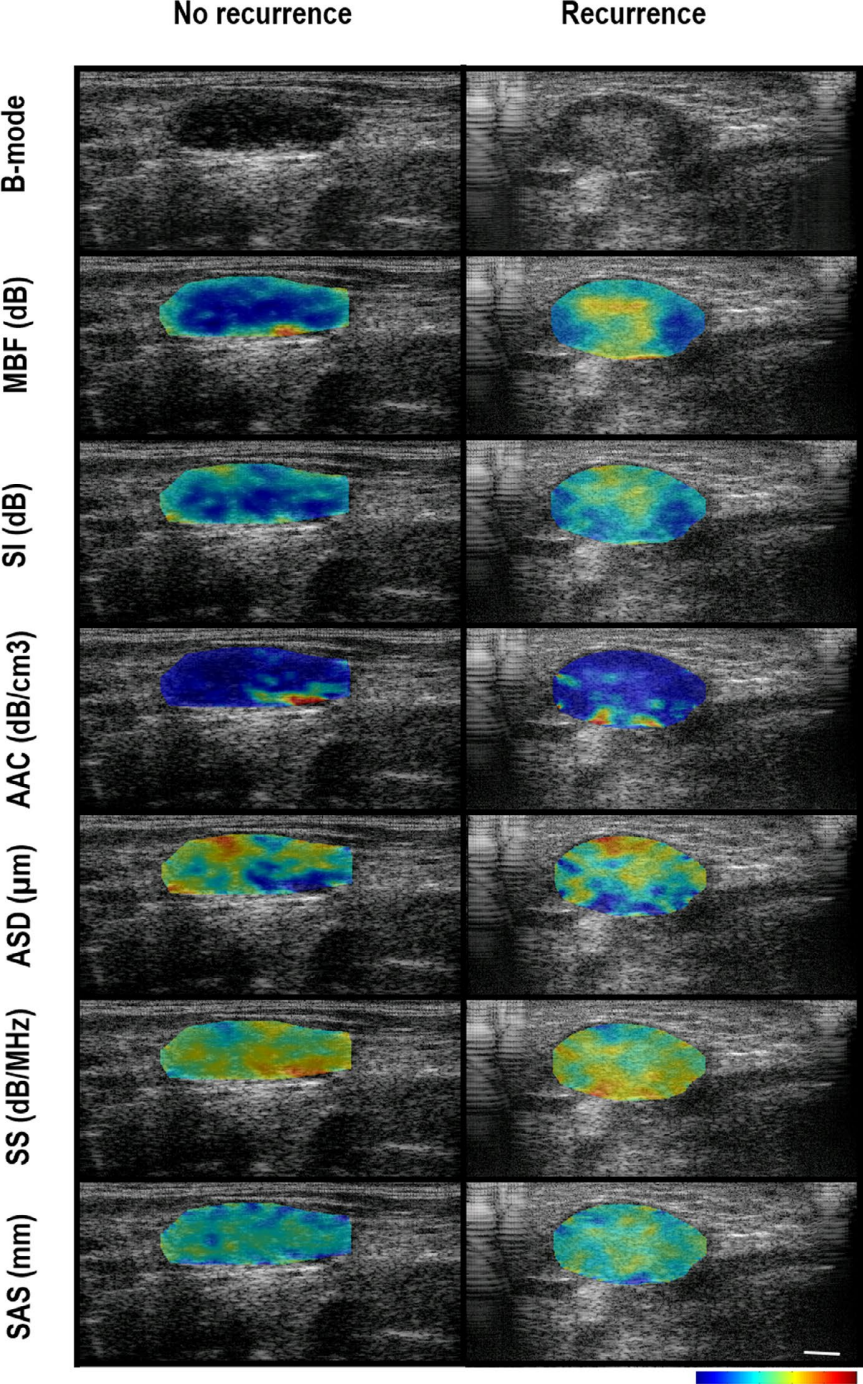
Representative ultrasound B‐mode images (upper row) with six spectral parametric maps from two patients—no recurrence (left panel) and recurrence (right panel). Parametric images from top to bottom represent overlays of the MBF, SI AAC, ASD, SS, and SAS parameters. The white scale bar (right lower corner) represents a length of 5 mm. The color bars present the range for MBF parameter of −10 dB to 25 dB, SI parameter of −10 dB to 60 dB, AAC parameter of 20 dB/cm‐MHz to 170 dB/cm‐MHz, ASD parameter of 1 µm to 200 µm, SS parameter of −8 dB/MHz to 22 dB/MHz, and SAS parameter of 0.2 mm to 2.5 mm
